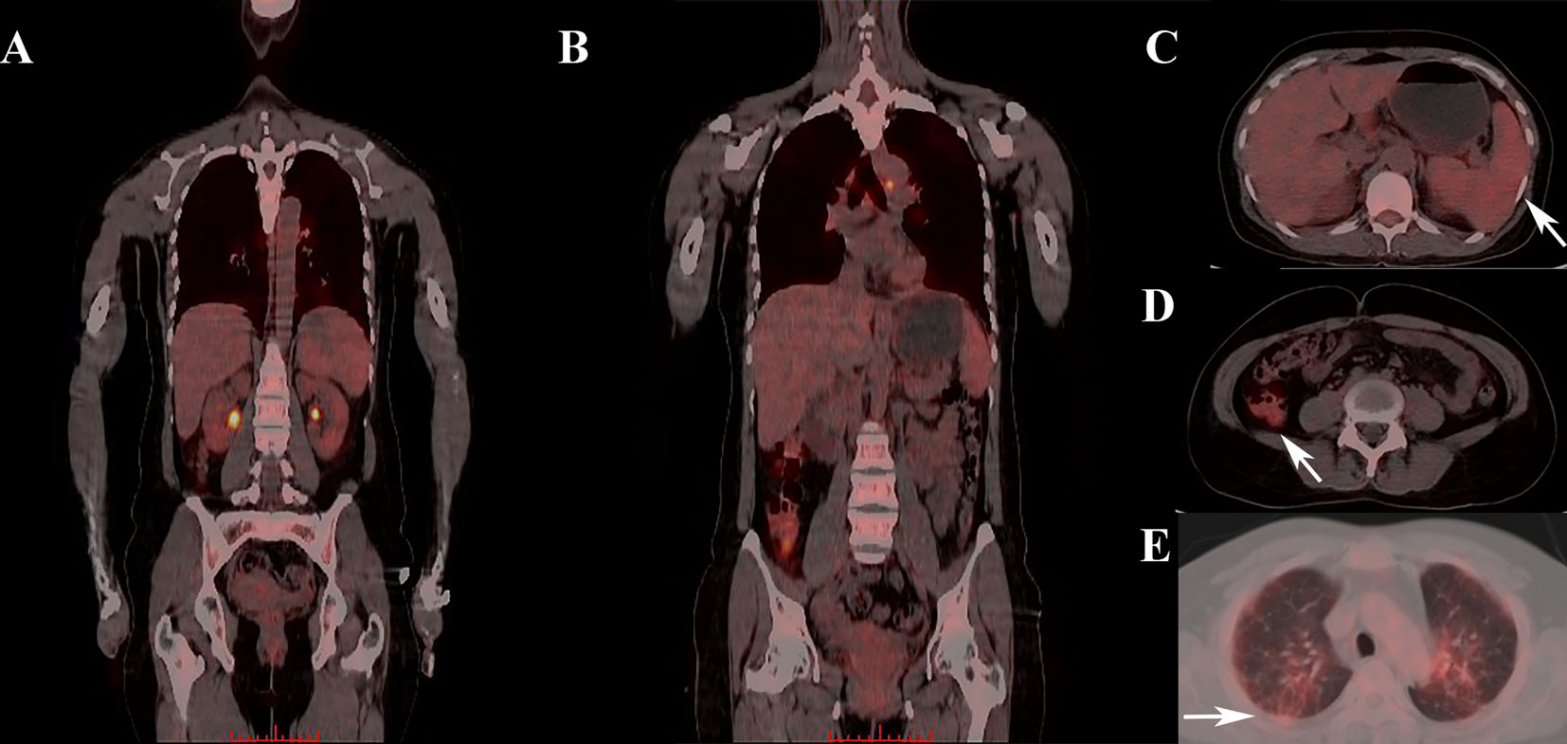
Abnormal FDG uptake in one IIM patient who was later diagnosed with sHLH. (A, B) Whole-body PET/CT scan showing abnormal FDG uptake in multiple organs. (C) Elevated splenic FDG uptake in PET/CT scan (where the arrow pointed). (D) Elevated colon FDG uptake in PET/CT scan (where the arrow pointed). (E) Elevated pulmonary FDG uptake in PET/CT scan (where the arrow pointed). FDG, Fluorodeoxyglucose; IIM,diopathic inflammatory myopathy; sHLH, Secondary hemophagocytic lymphohistiocytosis.
